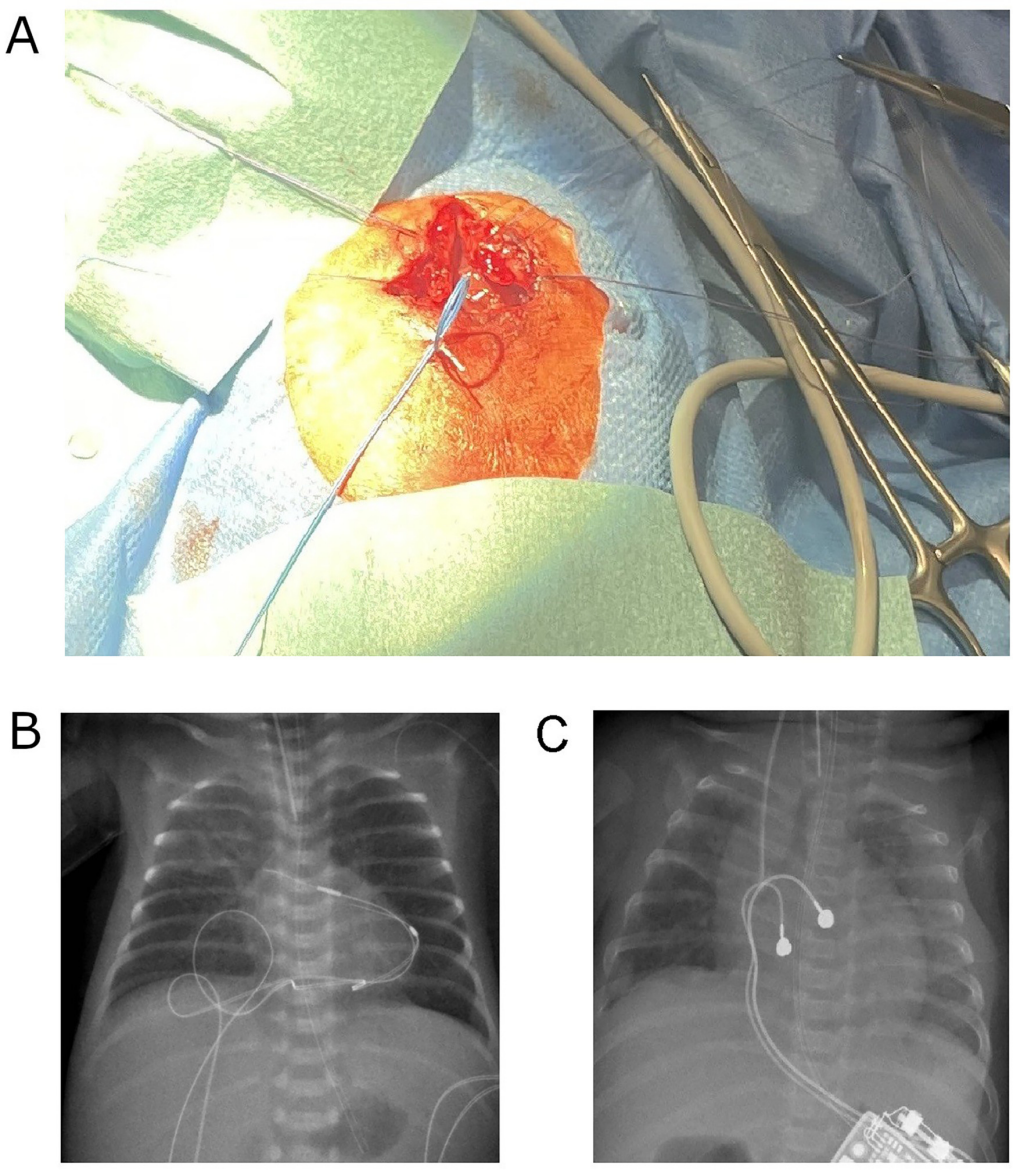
(A) Intraoperative image showing the placement of two epicardial pacing electrode pairs on the right ventricular surface (ventral and caudal sites) in the ELBW infant. (B) Postoperative chest and abdominal x-ray on day 5 of life showing the dual-site temporary pacing strategy, with two sets of epicardial pacing wires positioned at distinct ventral and caudal locations. (C) Postoperative chest and abdominal x-ray on day 90 of life demonstrating the permanent pacemaker generator positioned in the left upper abdominal quadrant and the bipolar lead attached to the right ventricular diaphragm and free wall.
